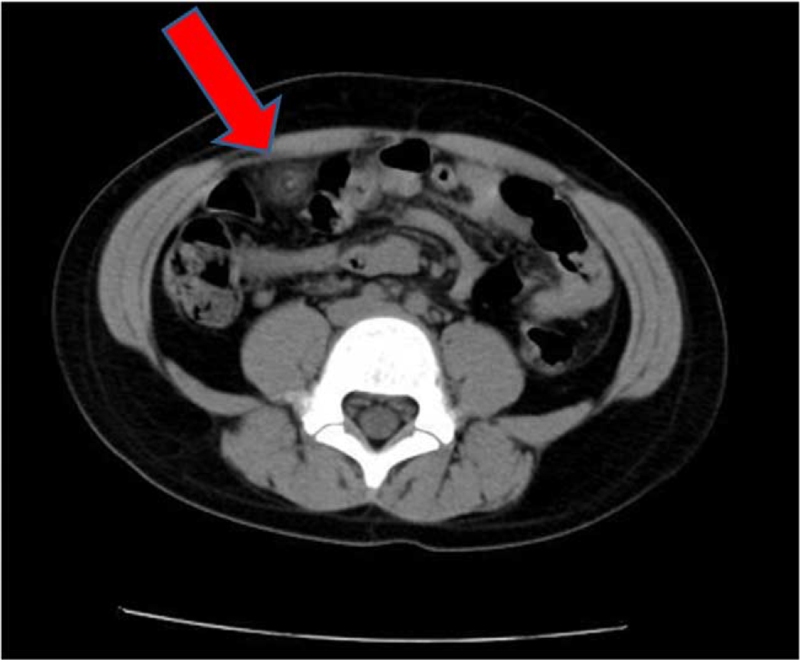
CT scan images of the omental torsion with concentric lines of fat and fibrous tissue surrounding the high density of central vessel at the right lower quadrant and iliac fossa (red arrow).
